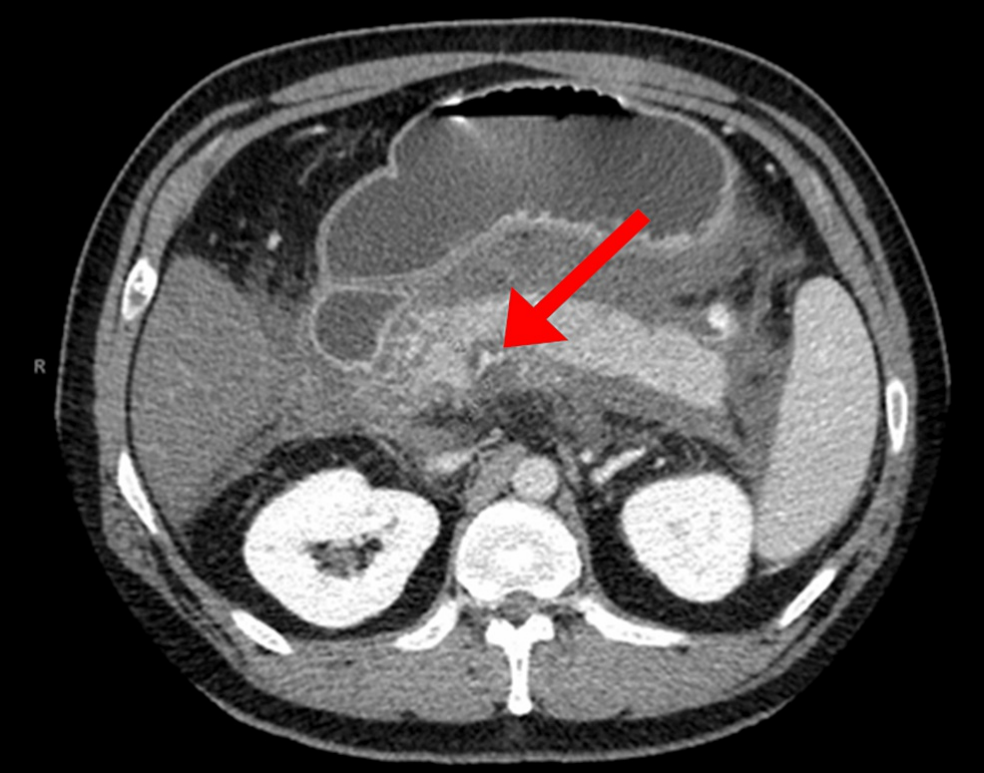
Computed Tomography Scan of Abdomen: Superior Mesenteric Vein Thrombosis (red arrow)
